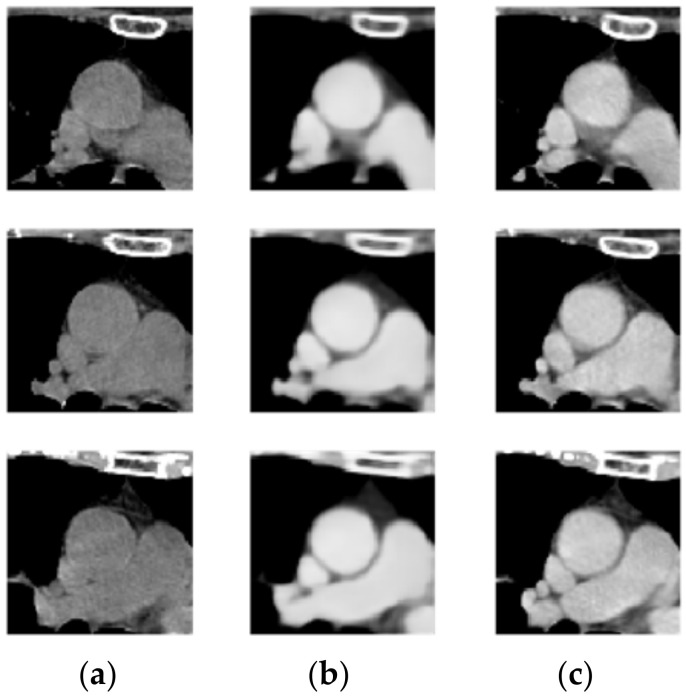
(a) Non-contrast-enhanced chest CT, (b) enhanced image, and (c) contrast CT image.
